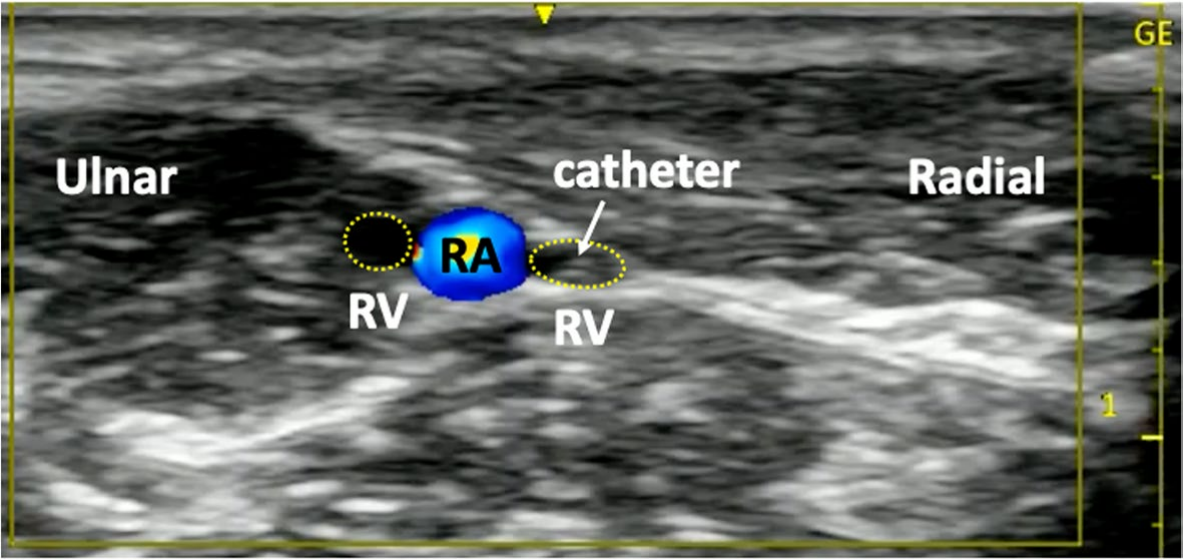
Ultrasound short-axis view of the left forearm in Case 1
